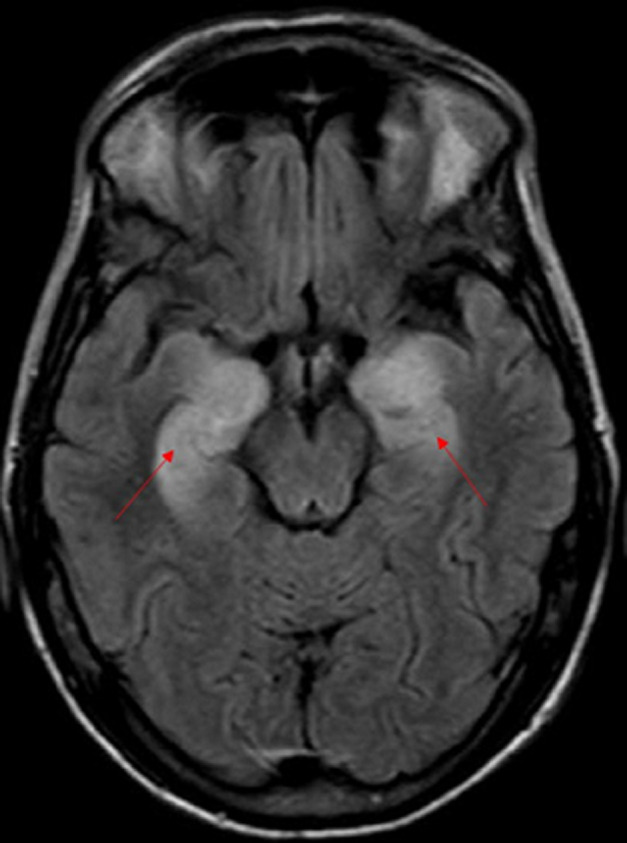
coupe axiale d’une IRM cérébrale montrant un hypersignal T2-FLAIR, en cortico-sous cortical temporal interne bilatéral
